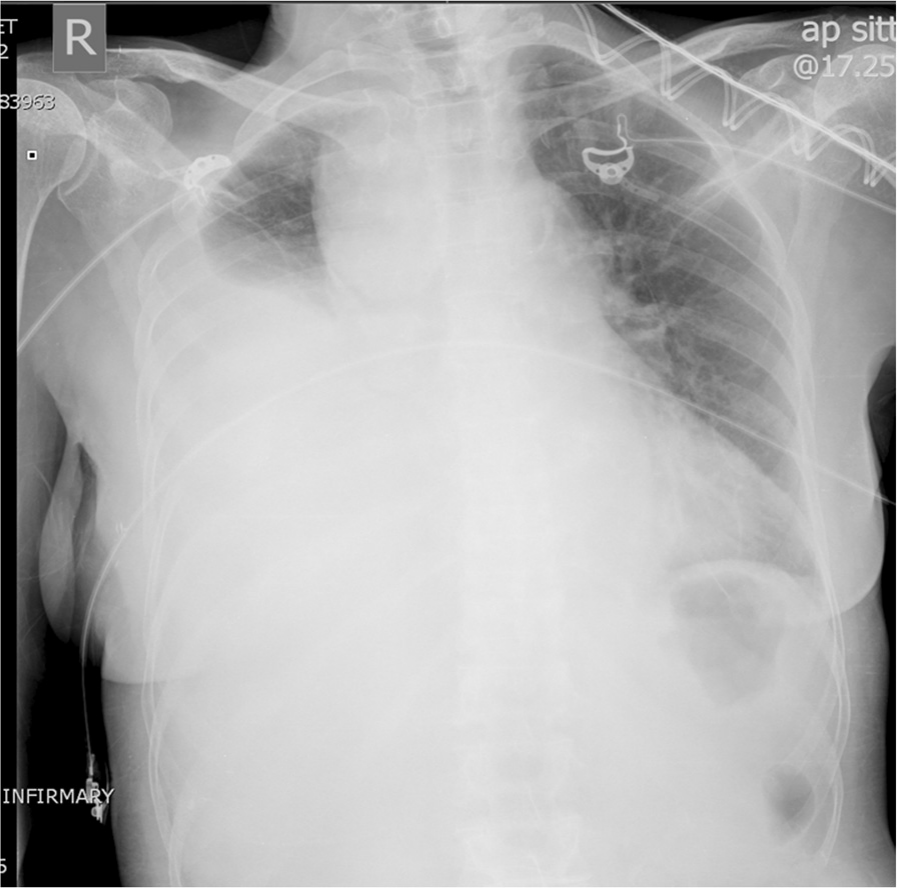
Chest X-ray shows massive right hemothorax, superior mediastinal widening and tracheal deviation to the left.
